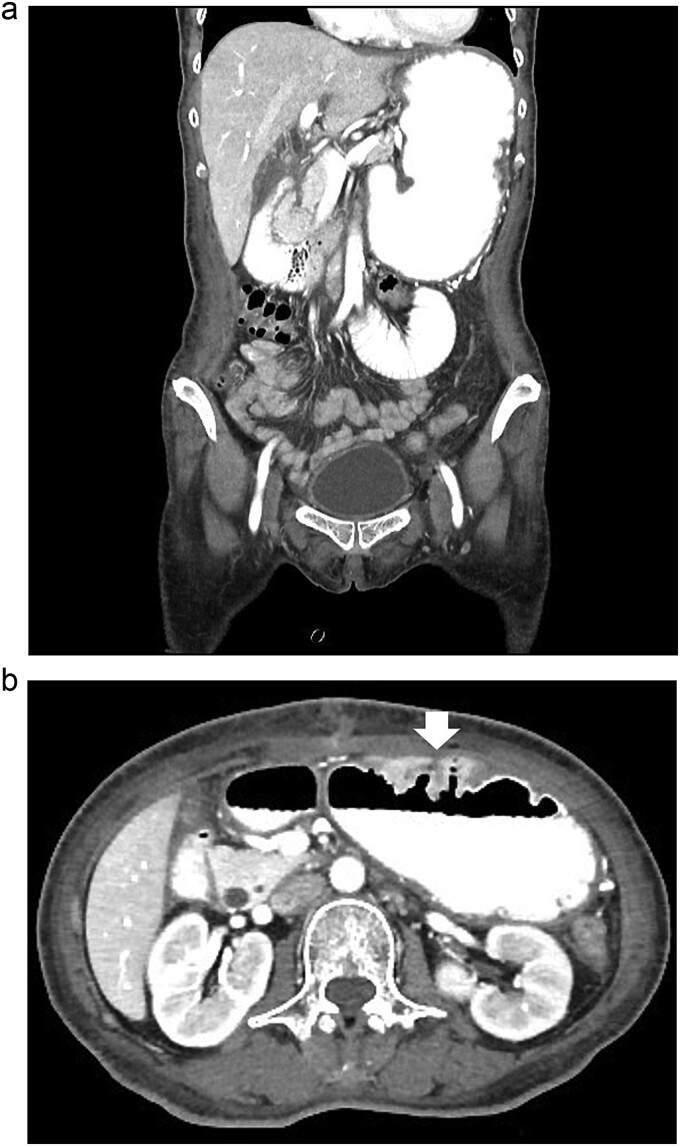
The CT scan of abdomen: (a) coronal view showed dilated stomach and afferent limb, (b) axial view showed gastrojejunostomy anastomosis angulation and kinking (arrow).
